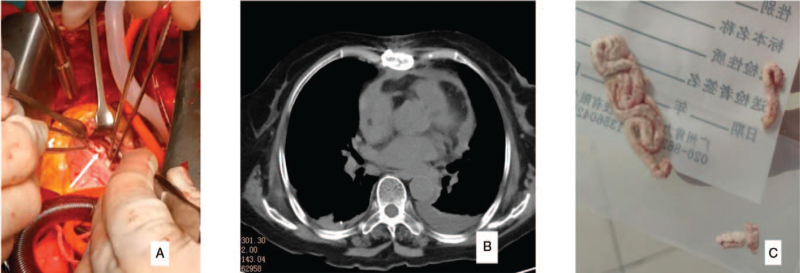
Intraoperative photos (arrows indicate bone cement) and postoperative CT scan of the chest, showing that the bone cement has been taken out; C is the bone cement taken out.
